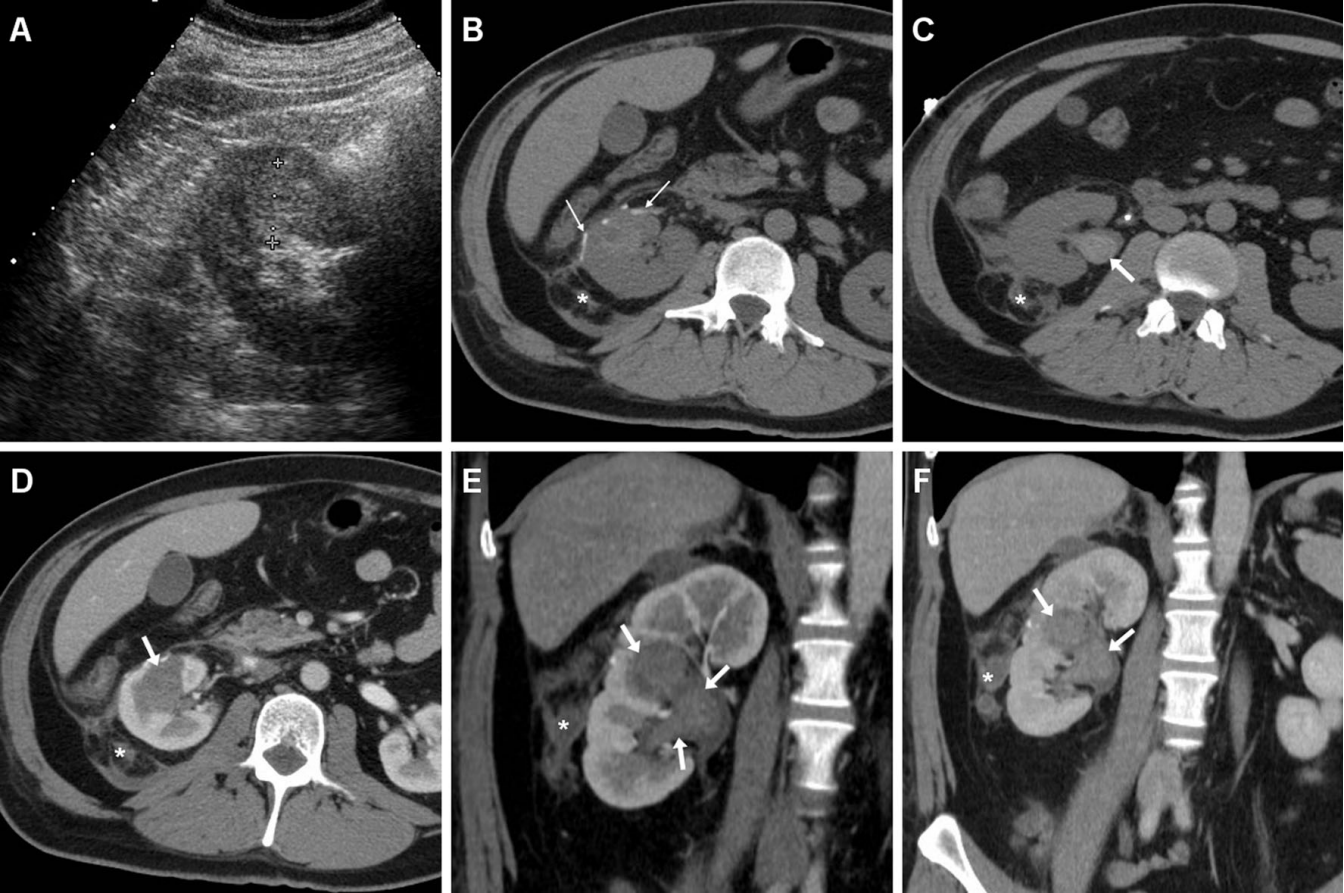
Gross haematuria with hypotension, moderate leucocytosis and blood loss (12 g/dL haemoglobin) in a 32-year-old man shortly after L-PN for a 2.5-cm, hyperechoic RCC (sonographic image in a). Unenhanced axial CT images (a, b) showed hyperattenuating linear structures (*thin arrows*) corresponding to surgical sutures along the ventral renal contour, minimal fluid and blood in the ipsilateral peri-renal space and retroperitoneal fasciae (*), hyperattenuating blood (*arrow*) in the renal pelvis. Corticomedullary (d, e) and nephrographic (f) phase images confirmed blood (*arrows*) occupying most of the renal collecting system. The lack of CM extravasation indicating active bleeding allowed conservative treatment including prolonged vesical catheterisation and lavage
